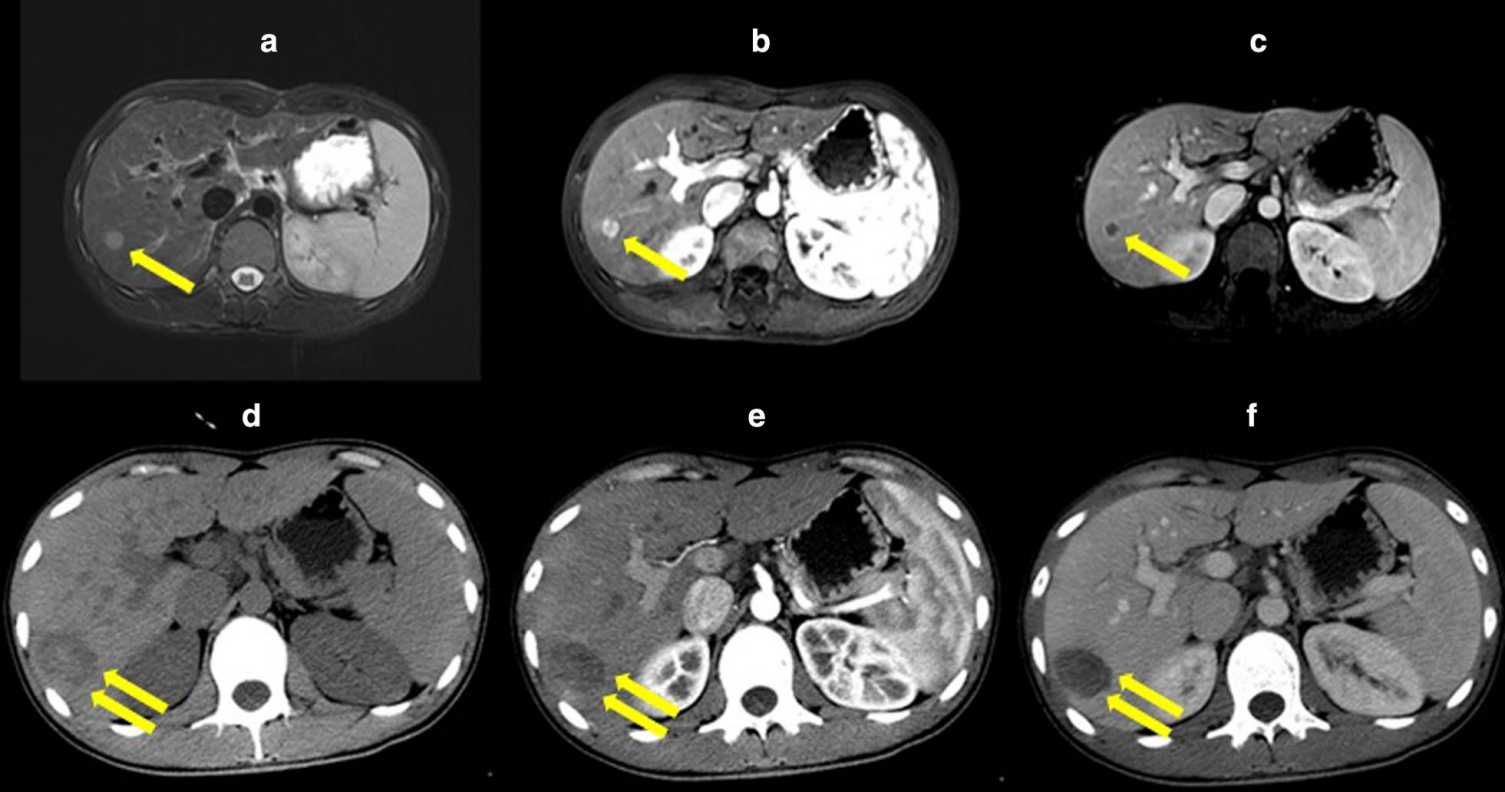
Comparison of pre-treatment and post-treatment tumor lesion images of a 46-year-old patient with hepatocellular carcinoma (HCC) and hepatitis B-related liver cirrhosis who underwent microwave ablation. Magnetic resonance images show a tumor with a small (15-mm) hyperintense nodule (a T2-weighted phase), intense arterial enhancement (b arterial phase), and enhancement recession (c portalvenous phase). Computed tomography images (d noncontrast-enhanced phase; e arterial phase; f portalvenous phase) obtained 2 days after treatment show no contrast enhancement inside or around the ablation zone
